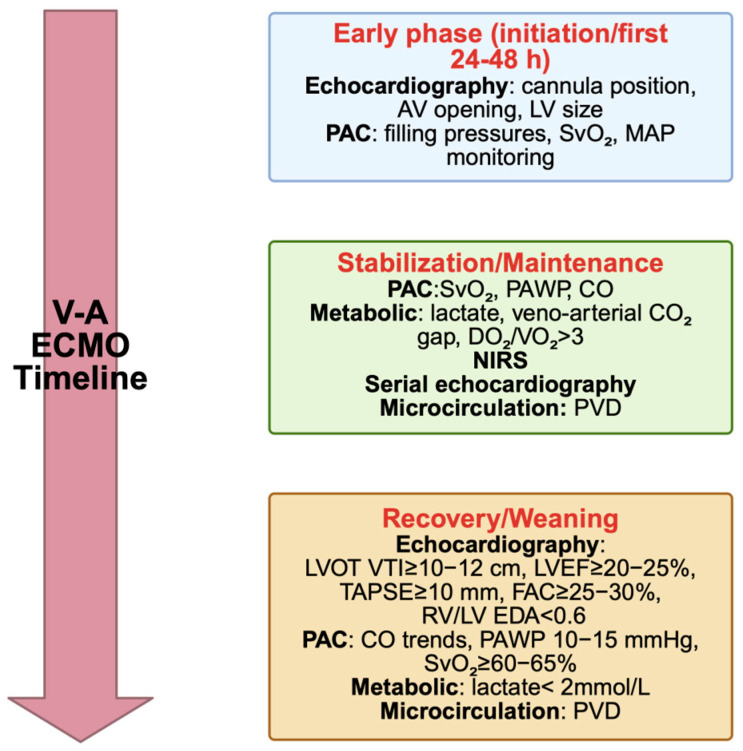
Time-dependent multiparametric hemodynamic monitoring in V-A ECMO. LVOT VTI: left ventricular outflow tract velocity time integral; LVEF: left ventricular ejection fraction; TAPSE: tricuspid annular plane systolic excursion; FAC: fractional area change; TDI S’: tissue Doppler imaging systolic velocity; RV/LV: right ventricle/left ventricle; PAC: pulmonary artery catheter; SvO_2_: mixed venous oxygen saturation; PAWP: pulmonary artery wedge pressure; CO: cardiac output; NIRS: near-infrared spectroscopy; PVD: perfused vessel density. Figure author generated using BioRender (49 Spadina Ave. Suite 200 Toronto ON M5V 2J1 Canada www.biorender.com).
